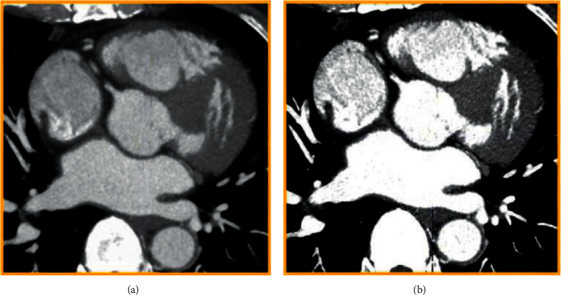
The effect of CTA data enhancement processing. (a) The original image; (b) the enhanced image.
